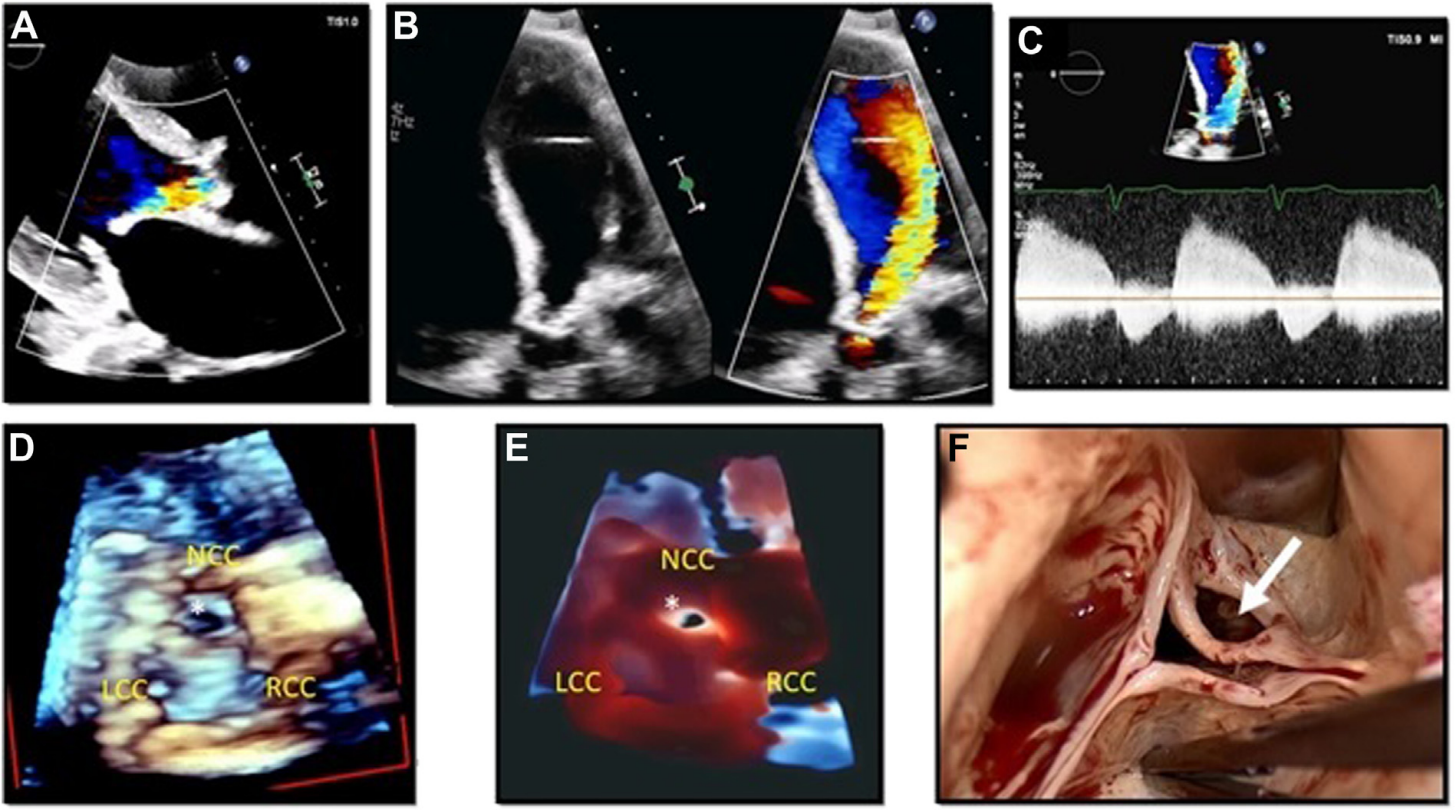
Visualization of a perforation on the AV NCC using 3D TI rendering on TTE. Panel A corresponds to a TTE PLAX view, where thickened AV leaflets and an eccentric jet of AR can be appreciated. Panel B shows thickened AV leaflets in a TTE apical 5-chamber view *(left image)*, showing with color Doppler *(right image)* the presence of a broad jet of severe AR directed toward the inferolateral LV wall. Panel C corresponds to an AV CW Doppler consistent with severe AR. Panel D shows a 3D zoom rendering of the AV. There is a defect on the NCC *(white asterisk)*, which is better depicted with the use of TI rendering as shown in panel E. Panel F corresponds to the surgical findings, showing the exact correlation with the TTE findings *(white arrow)*. *LCC*, Left coronary cusp; *RCC*, right coronary cusp.
